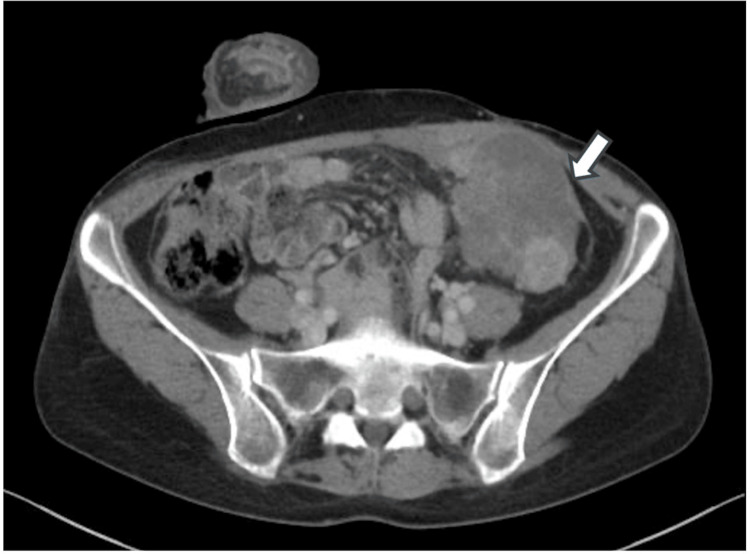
CT findings after 10 cycles of neoadjuvant chemotherapy with FOLFOX After neoadjuvant chemotherapy with FOLFOX, the tumor shrank significantly from 9.7 cm to 7 cm in diameter (arrow). Although abdominal wall invasion persisted, the area of tumor invasion was markedly reduced.
